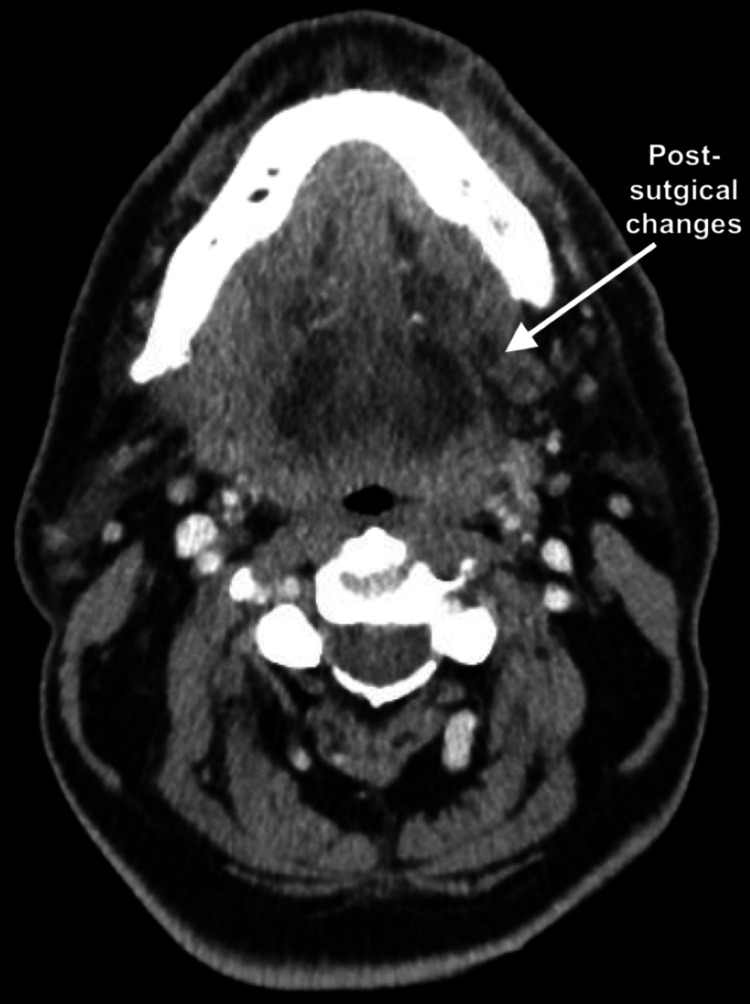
Post-operative day eight CT soft tissue neck Postsurgical changes in submandibular spaces, especially on the left side (white arrow). No evidence of discrete abscess collection
